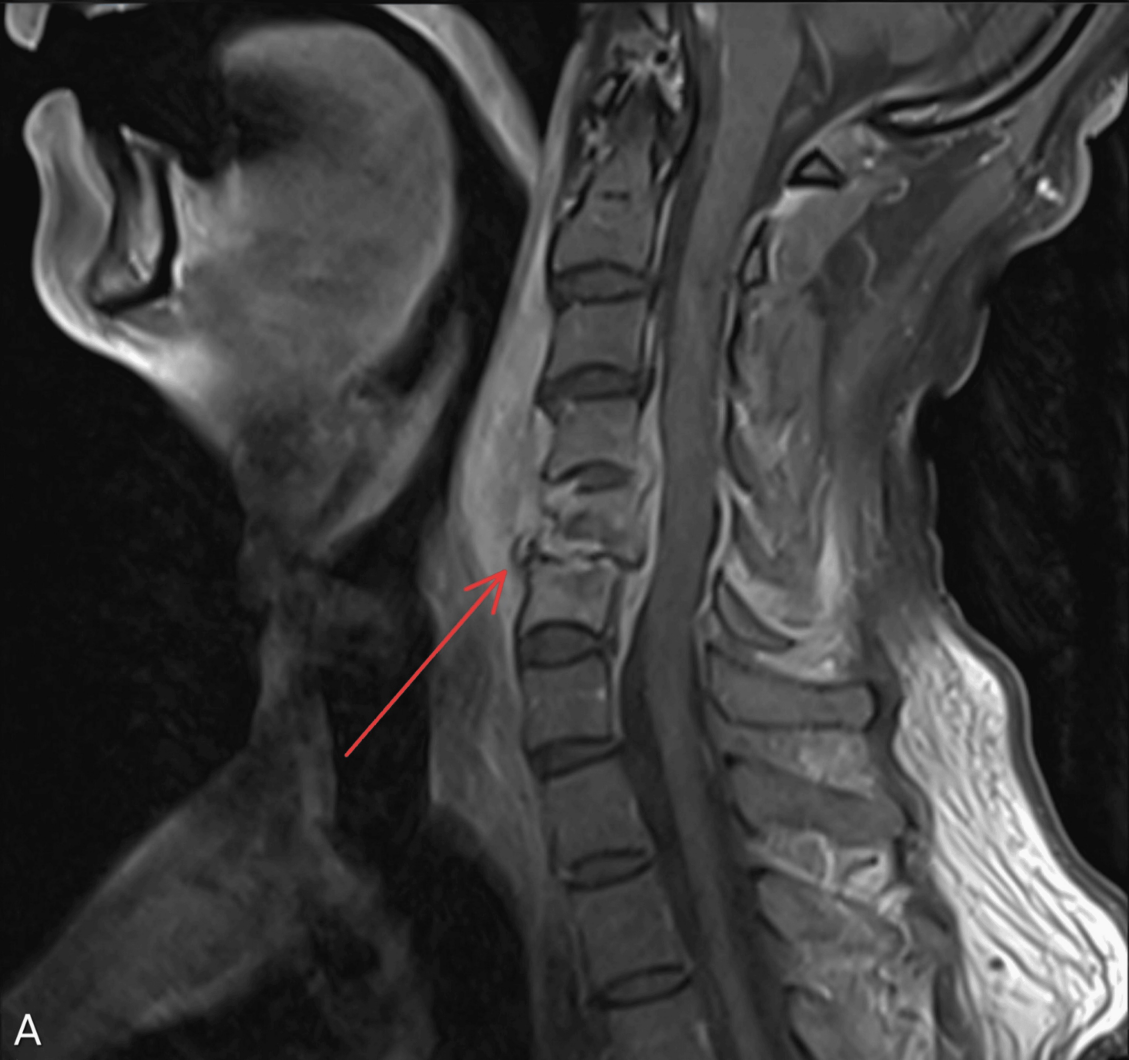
MRI of the cervical spine with the red arrow showing osteomyelitis at C5-C6 with evolving infectious process in the adjacent tissue
